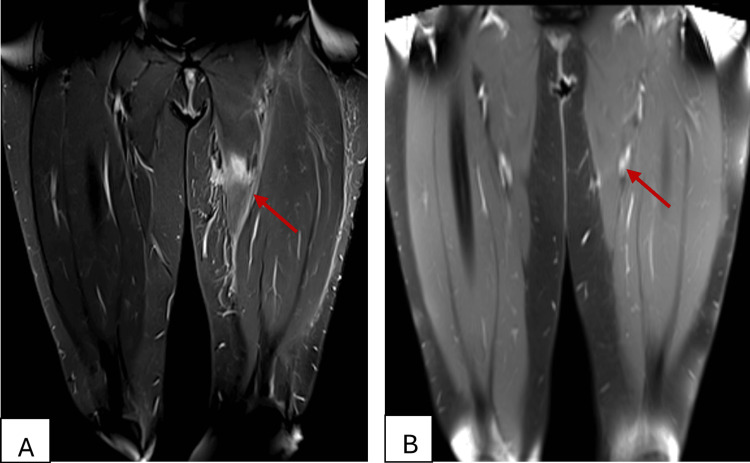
A: Coronal MRI of the left thigh (T2/STIR with contrast) demonstrating hyperintensity and enhancement in the quadriceps and adductor muscles without abscess or collection. B: Follow-up coronal MRI of the left thigh (T2/STIR with contrast) at 6 months showing complete resolution of muscle edema STIR, short-tau inversion recovery; MRI, magnetic resonance imaging.
